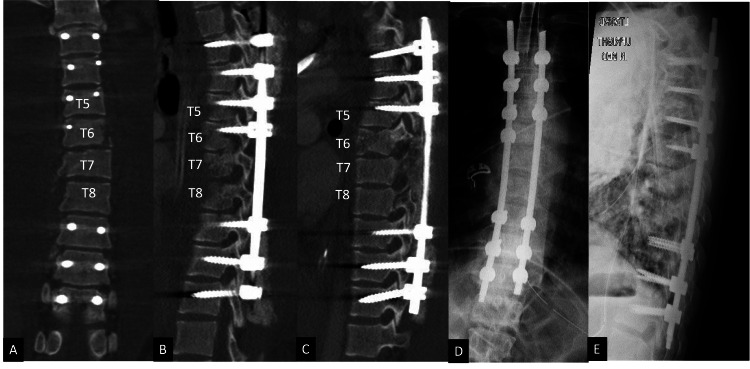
Immediate post-operative computed tomography and plain film radiographs of the thoracic spine demonstrating complete reduction of the T6-T7 spondyloptosis with the near-anatomic restoration of vertebral body alignment, bilateral facet joints, and the spinal canal. (A) Post-operative coronal computed tomography scan. (B) Post-operative right paramedian sagittal computed tomography scan. (C) Post-operative left paramedian sagittal computed tomography scan. (D) Post-operative upright anteroposterior plain film. (E) Post-operative sagittal plain film. T5, T6, T7, and T8 indicate thoracic vertebral levels.
